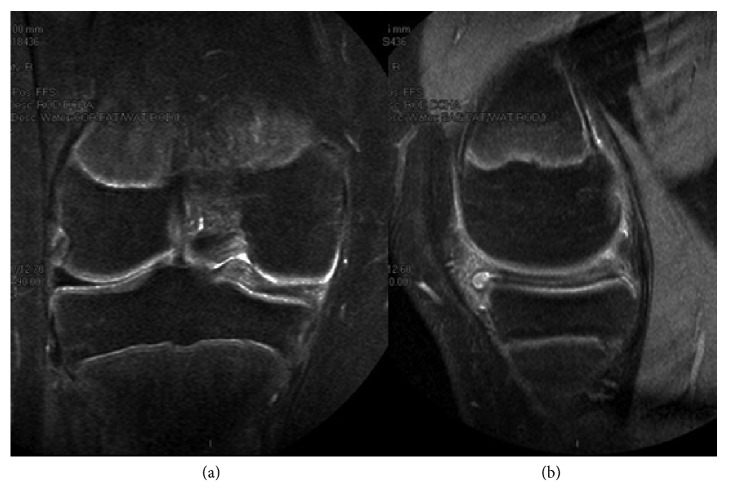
MRI of the right knee. (a) Coronal view showing the upper side of the medial meniscus folded in the intercondylar notch. (b) Sagittal view demonstrating the horizontal tear.
